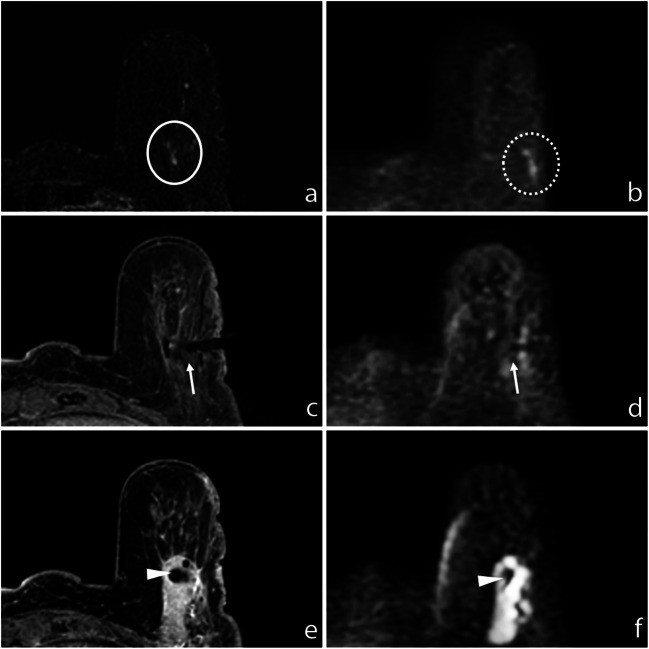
a Subtracted DCE 3D-THRIVE showing a non-mass lesion in the inferior sagittal plane of the left breast of a 71-year-old woman (circle). b DWI (*b* = 1300 s/mm^2^) showing hyperintensity of the enhancing lesion, slightly lateral-sided than real location due to spatial distortion artefacts (dotted circle). c T1-WI FS GRE showing the proper position of the biopsy device (arrow); the end of needle is well recognizable at (d) DWI (*b* = 1300 s/mm^2^, arrow). e T1-WI FS GRE showing the correct deployment of the site marker (arrowhead) that is also recognizable at (f) final DWI (*b* = 1300 s/mm^2^, arrowhead)
